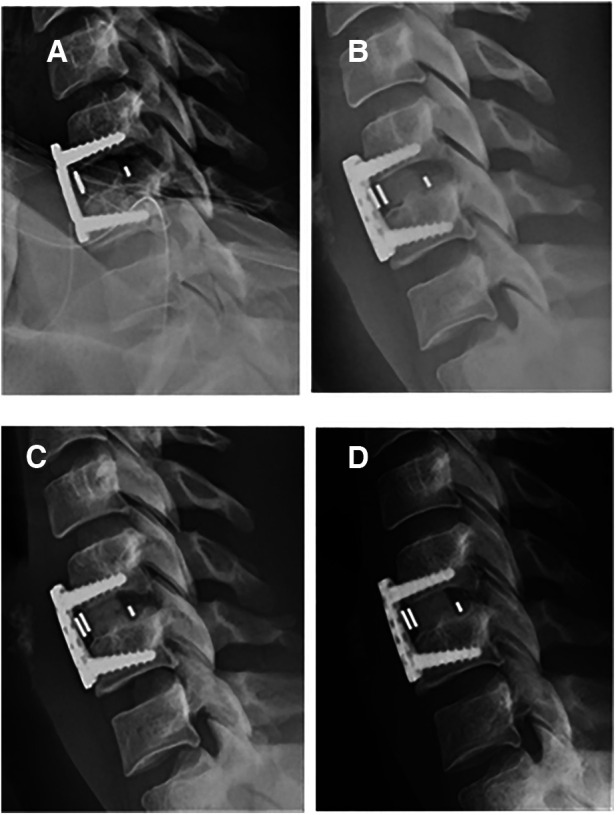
One-level ACDF arthrodesis using BIOBank allograft on a 37-year-old female at C5C6. x-Ray lateral view at immediate post-operative (A), 1 month postoperative (B), 4.5 months postoperative with visible fusion (C) and 1 year postoperative (D).
